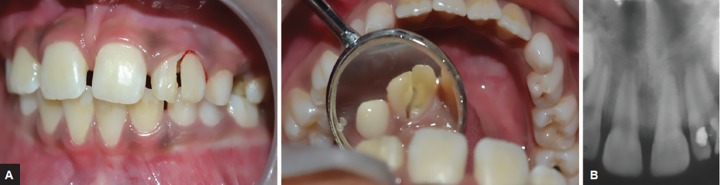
(A) Intraoral photographs: the fracture line extending from the incisor edge to below the free gingival margin involving both labial and palatal surfaces of maxillary left lateral incisor. (B) Intraoral periapical radiograph: the fracture line extending at the level of crest of alveolar bone
